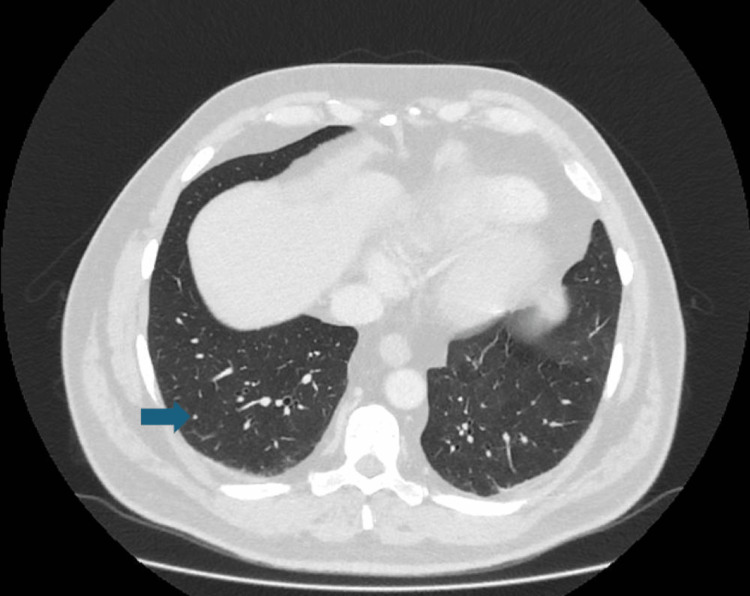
CT of the thorax (transverse view) showing a 3 cm lung mass in the right lower lobe.
